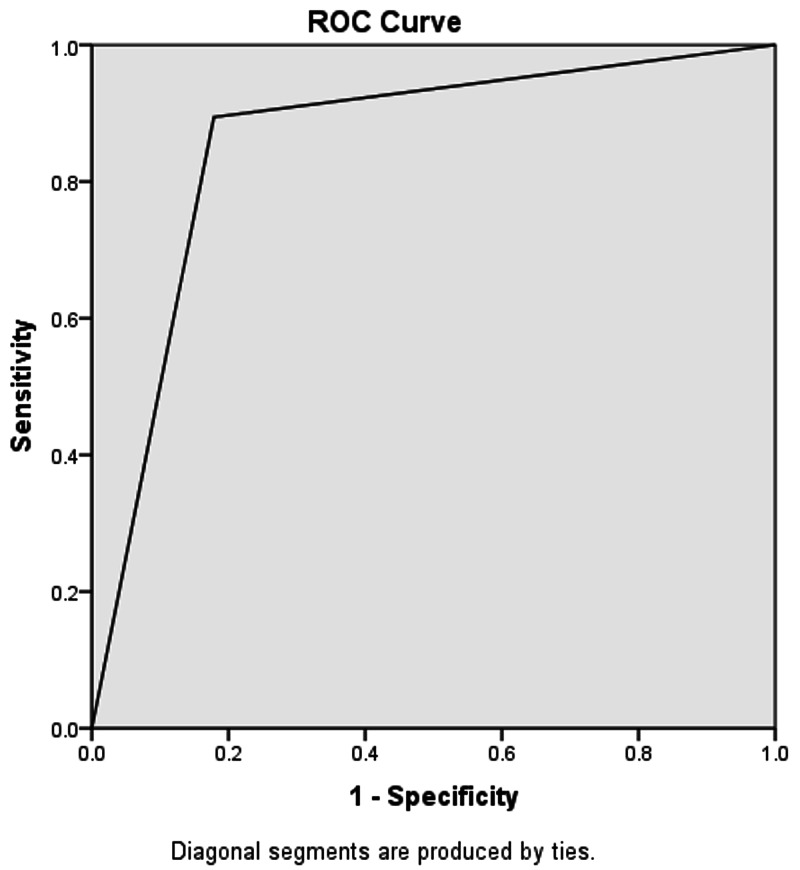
Area under the curve for the ultrasound scan = 0.858
